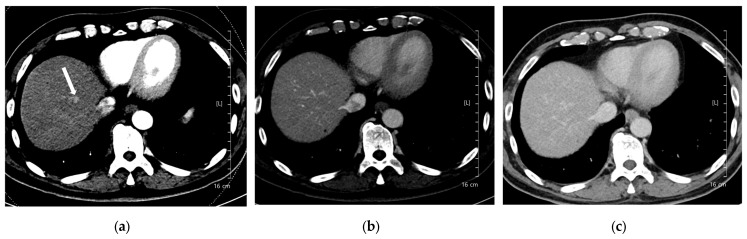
A 55-year-old man with hepatocellular carcinoma (HCC) on magnetic resonance imaging, characterized by diffusion restriction, high signal intensity on T2-weighted images, and washout. CT was performed using high-concentration iodine contrast material (total iodine dose, 584.14 mg I/kg). Overall image quality in the arterial and portal phases was acceptable. (a) A 7-mm enhancing nodule (arrow) is visible on the arterial phase at 100 kVp; (b,c) The nodule is inconspicuous on the portal and delayed phases. Both reviewers classified the lesion as benign (LI-RADS category 3).
